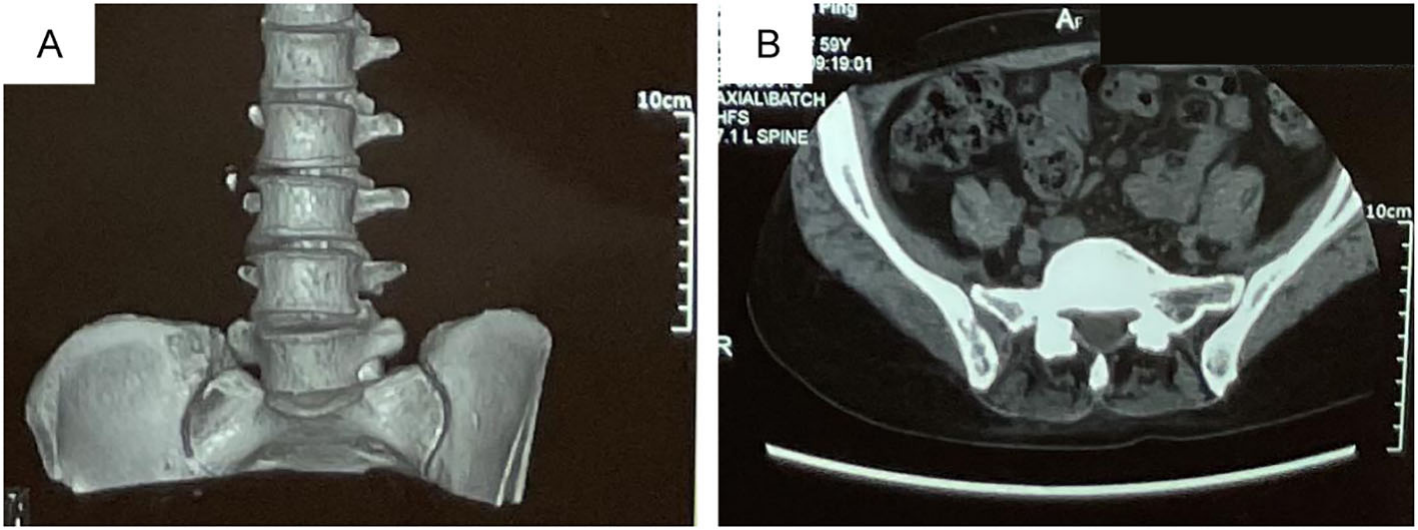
The right sacroiliac joint bone destruction with soft tissue mass (malignant?) was found by lumbar computed tomography (CT) scan on May, 11, 2023. (A) Three-dimensional reconstruction of the right sacroiliac joint. (B) Coronal CT scan of the right sacroiliac joint. The patient, a 60-year-old female with no history of smoking, had no prior medical conditions, with a chief complaint of the pain in the right lumbosacral region and was found the right sacroiliac joint bone destruction with soft tissue mass (malignant?) by lumbar computed tomography (CT) scan on May, 11, 2023 (A, B).
